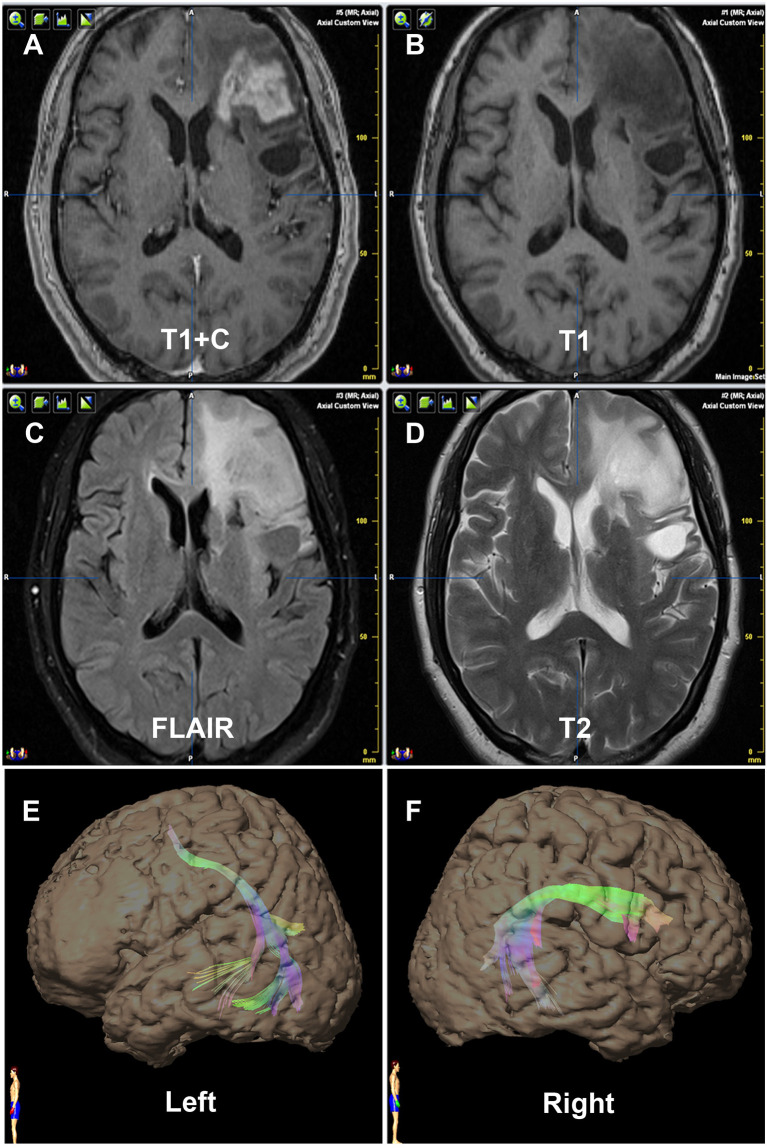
Contrast of bilateral AF by DTT of Patient No. 1. Panels (A–D) are preoperative MRI T1+C, T1, FLAIR, and T2, respectively, on one same transverse slice of Patient No. 1, who was diagnosed as recurrent astrocytoma in site in Broca’s area. Panels (E, F) show that the relative more fiber number and volume of AF are on the right hemisphere, which are in concordance with the language dominant side of the Wada test.
